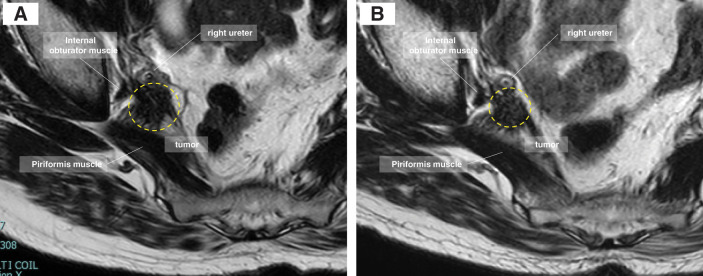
T2-weighted MRI reveals a tumor (dotted circle) with irregular borders and invasion of the right ureter, internal obturator muscle, internal iliac artery, and vein in the right lateral pelvic area. (A) Before pre-CRT. (B) After pre-CRT. pre-CRT, preoperative long-course chemoradiotherapy
